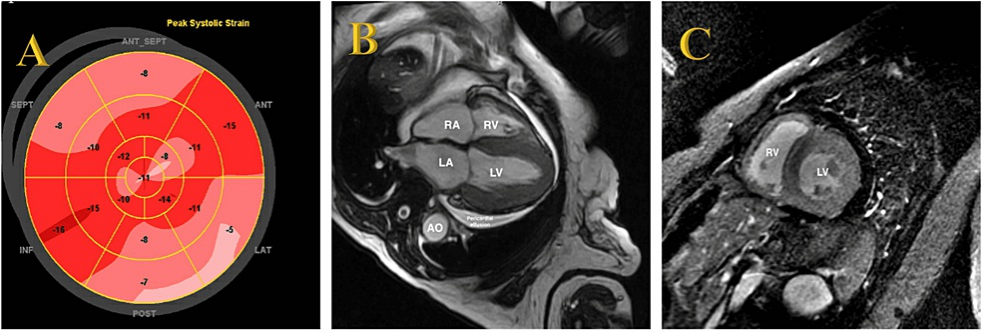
Transthoracic echocardiogram strain imaging and cardiac magnetic resonance image (CMRI). A. Apical sparing of longitudinal systolic strain (GLS -13.2%) consistent with cardiac amyloidosis. B. SSFP: Steady state free precession sequence demonstrating bi-atrial dilation and thickened left ventricular wall with moderate-sized pericardial effusion (LV: Left ventricle, RV: Right ventricle, LA: Left atrium, RA: Right atrium, Ao: Thoracic aorta). C. Look-Locker inversion recovery sequences with Late Gadolinium Enhancement (LGE) demonstrating diffuse dense circumferential and transmural left and right ventricular enhancement and Query Amyloid Late Enhancement (QALE) score of 18 most consistent with cardiac transthyretin amyloidosis [>13 scores, 82% sensitive, 76% specific for differentiating ATTR and AL]. (LV: Left ventricle, RV: Right ventricle).
